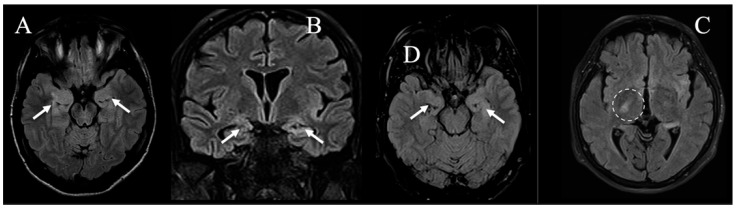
The most relevant FLAIR images at the first time-point MRI examination (A–C) in patients with bilateral mesial-temporal signal abnormalities associated with the distant primary alteration (respectively, cases #1, #4, and #5, *white arrows*), and (D) in a single patient with subtle changes in the internal capsule contralateral to the distant primary alteration (namely case #2, *dashed line*).
